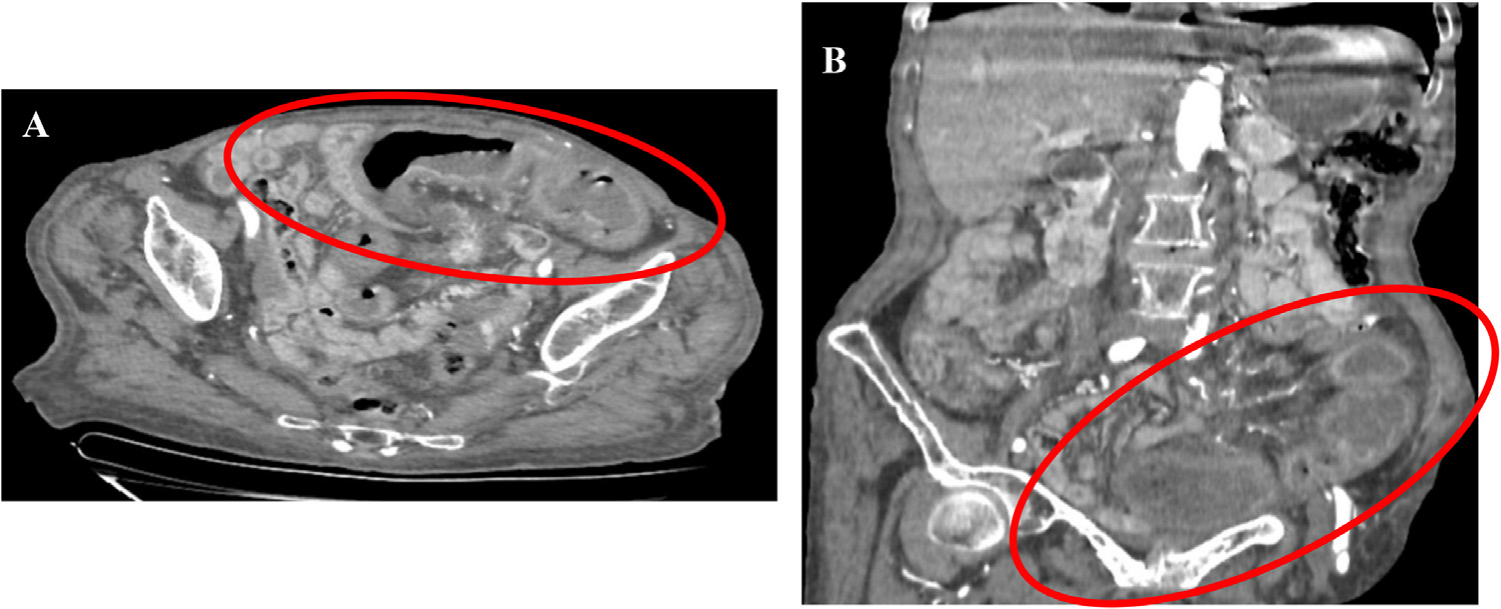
Axial enhanced abdominal CT scan (A) and coronal reformation (B) showing imaging features of subacute intestinal ischemia (red circle).
